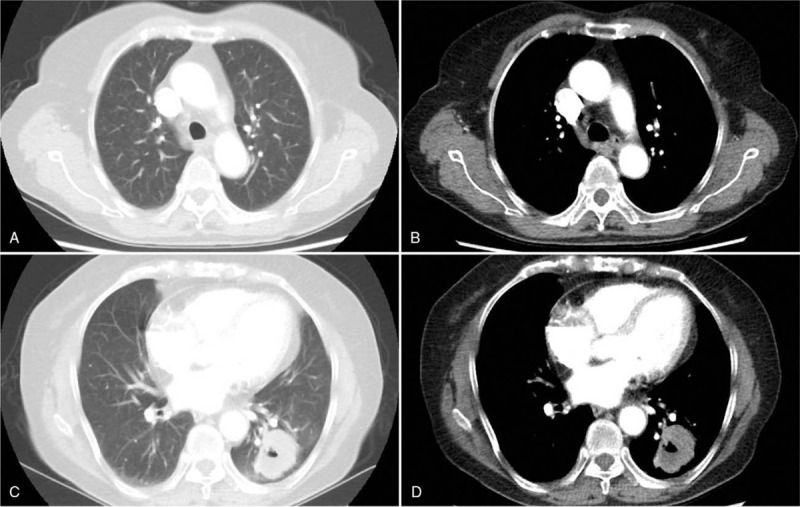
Mediastinal lymph node enlargement in the pulmonary window (A) and mediastinal window(B); a 41 × 38 mm mass shadow with a cavity of the left inferior pulmonary lobe in the pulmonary window (C) and mediastinal window (D) at the enhanced chest-CT.
